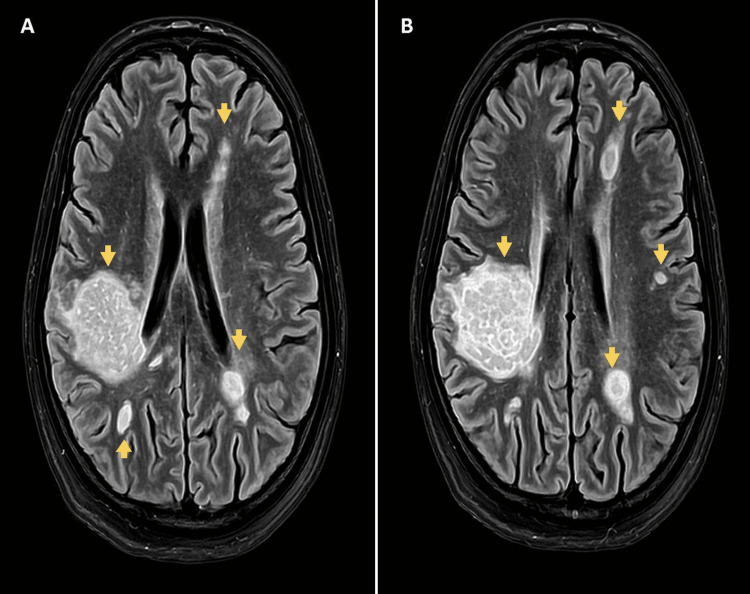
Axial brain MRI images (A and B) demonstrating cerebral abscesses (arrows) MRI, magnetic resonance imaging.
